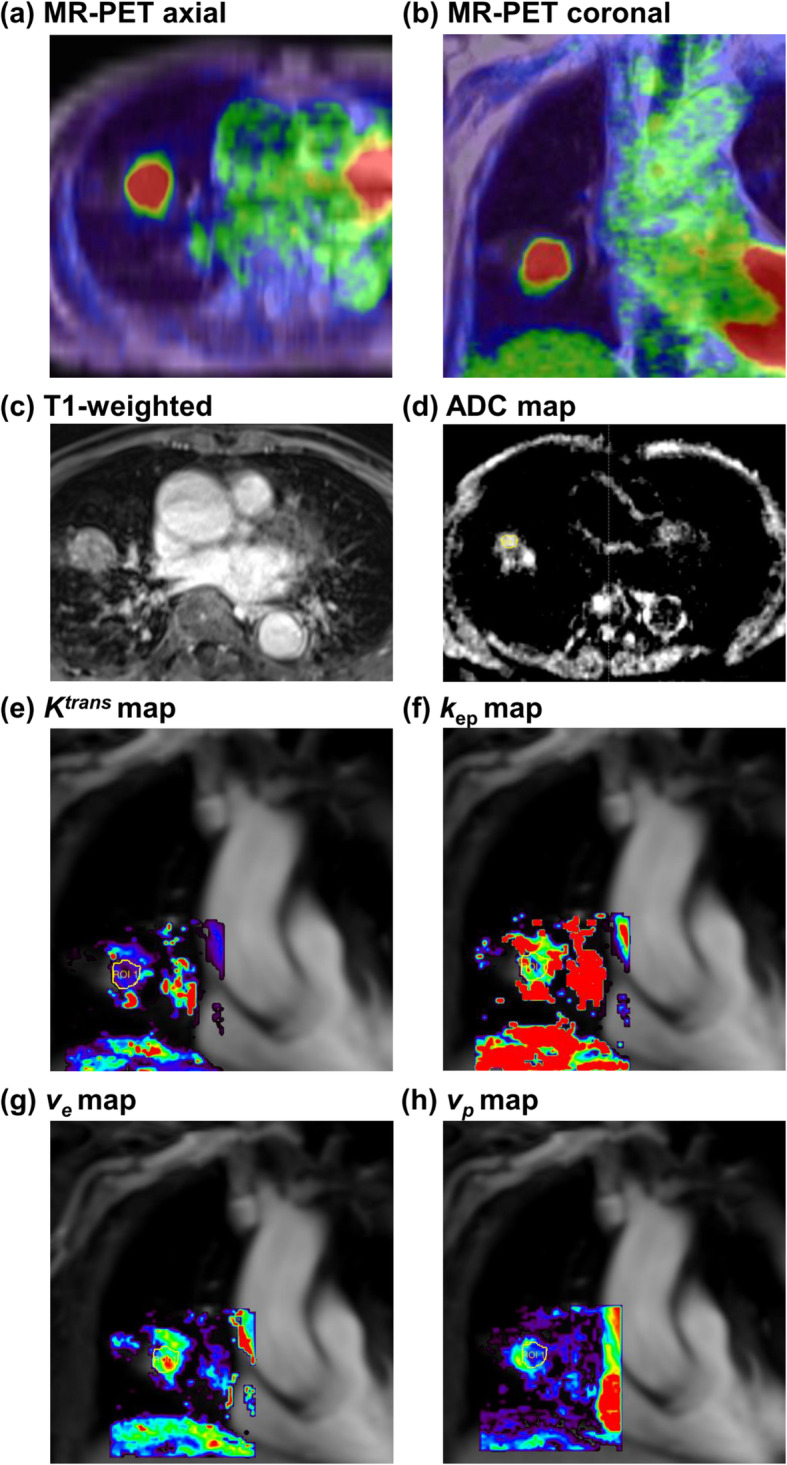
Dynamic contrast-enhanced integrated magnetic resonance-positron emission tomography parameters obtained at diagnosis in a patient with right lower lung adenocarcinoma (cT2aN0M0, stage IB). Measured magnetic resonance-positron emission tomography (MR-PET) parameters were as follows: (a, b) SUV_max_, 11; (c) tumor size, 3.0 cm (by T1-weighted post contrast); (d) ADC, 1025 (10^− 6^ mm^2^/s); (e) *K*^trans^, 110 (10^− 3^/min); (f) *k*_ep_, 598 (10^− 3^/min); (g) *v*_*e*_, 202 (10^− 3^); (h) *v*_*p*_, 107 (10^− 3^); and iAUC_60_, 253 (10^− 3^). Measured serum angiogenesis-related biomarkers included VEGF-A (119 pg/mL), angiopoietin-1 (46 ng/mL), angiopoietin-2 (128 pg/mL), and angiogenin (632 ng/mL). Due to medically inoperable status, the patient underwent stereotactic body radiation therapy. The patient achieved a complete response and remained disease-free for 48 months
